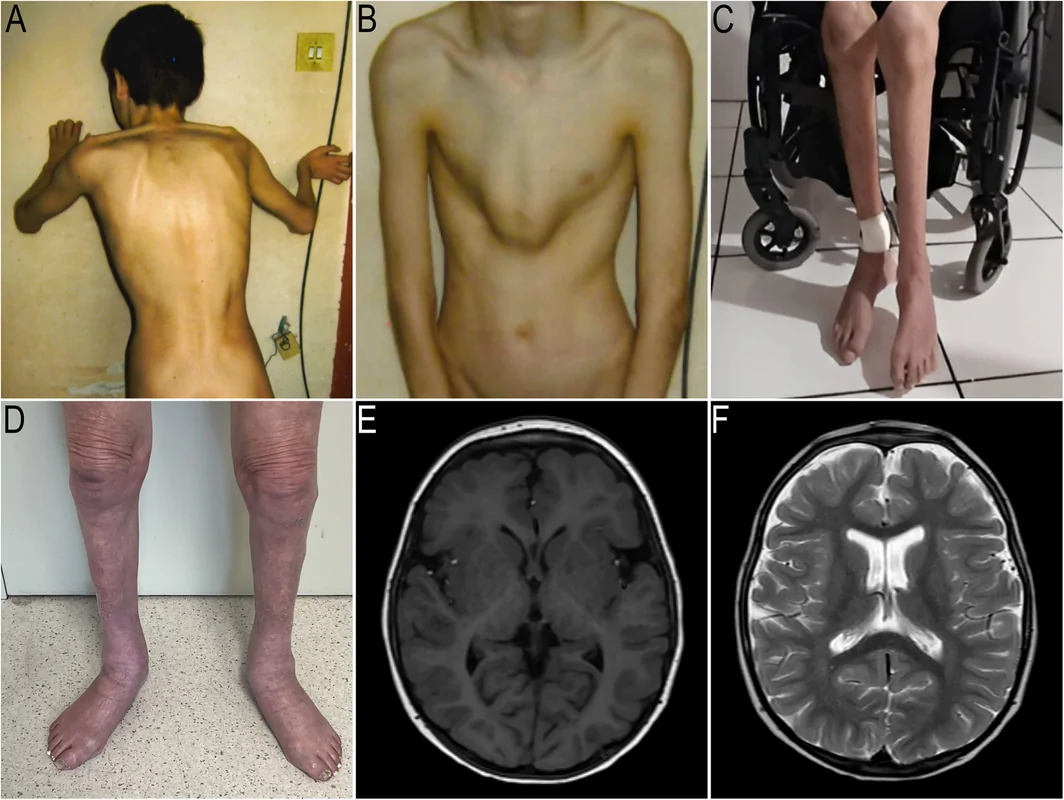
Clinical and neuroimaging features of patients with monoallelic *POLR3A* variants. (A, B) Muscle atrophy, scapula alata, kyphoscoliosis and *pectus carinatum* in patient H‐II.1 at age 12 years. (C) Muscle atrophy in lower limbs in patient D‐II.1 at age 19 years. (D) Muscle atrophy in the lower limbs in patient A‐I.1 at age 78 years. (E, F) Axial T1‐ and T2‐weighted brain MRI images of patient E‐II.1 at age 5 years showing normal white matter signal intensity for this age. MRI = magnetic resonance imaging. [Color figure can be viewed at www.annalsofneurology.org]
